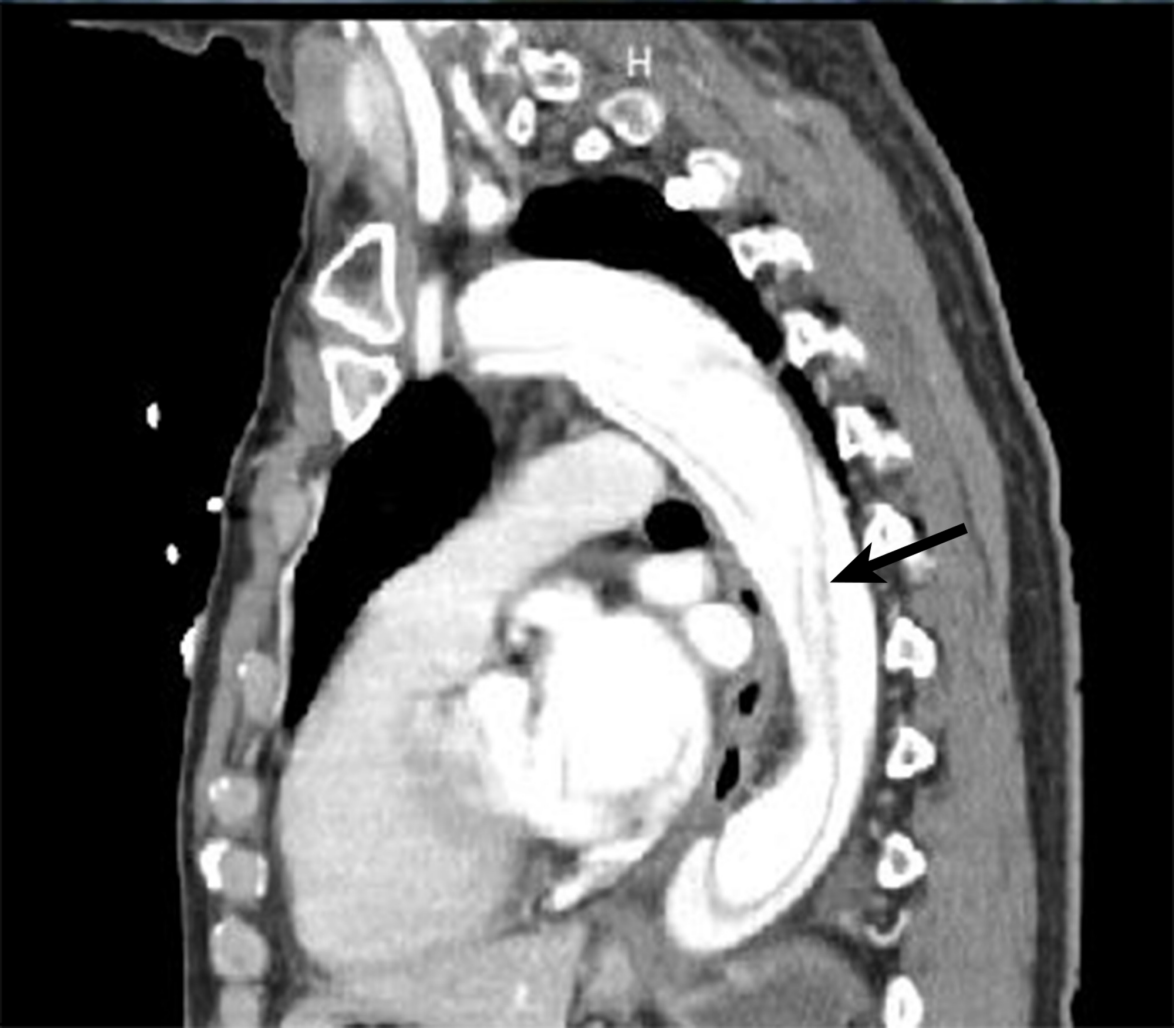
CTA showing type B AD with arch involvement
